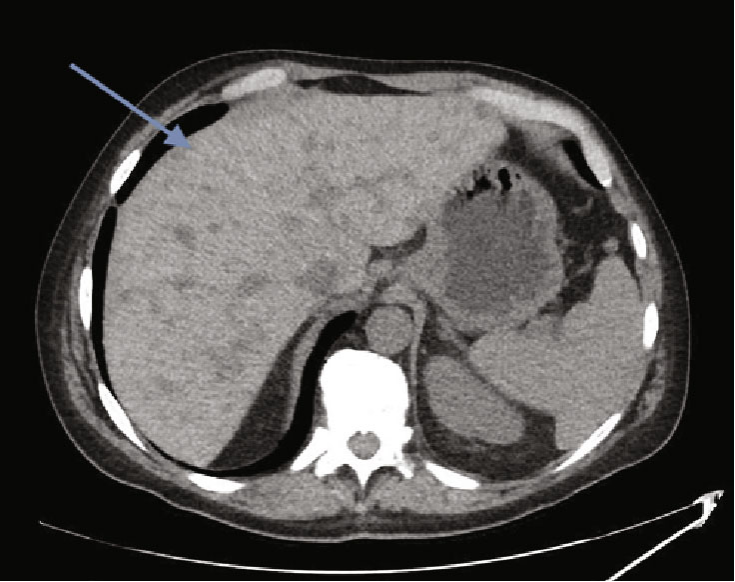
CT abdomen and pelvis was performed 2 months after starting chemotherapy which demonstrated a slight decrease in the size of hepatic metastatic lesions, which may represent a partial response to therapy (blue arrow).
